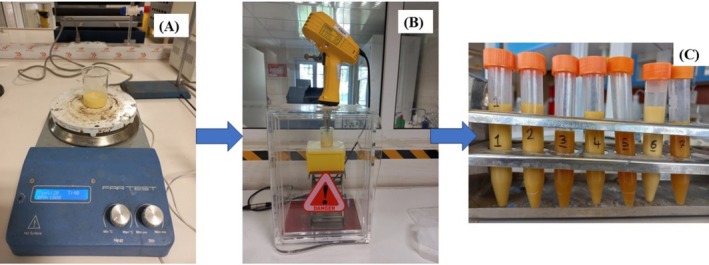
Preparation of LGEO nanoemulsion (A) mixture of raw material by a magnetic stirrer, (B) ultrasonication of NE, and (C) prepared NE samples.
